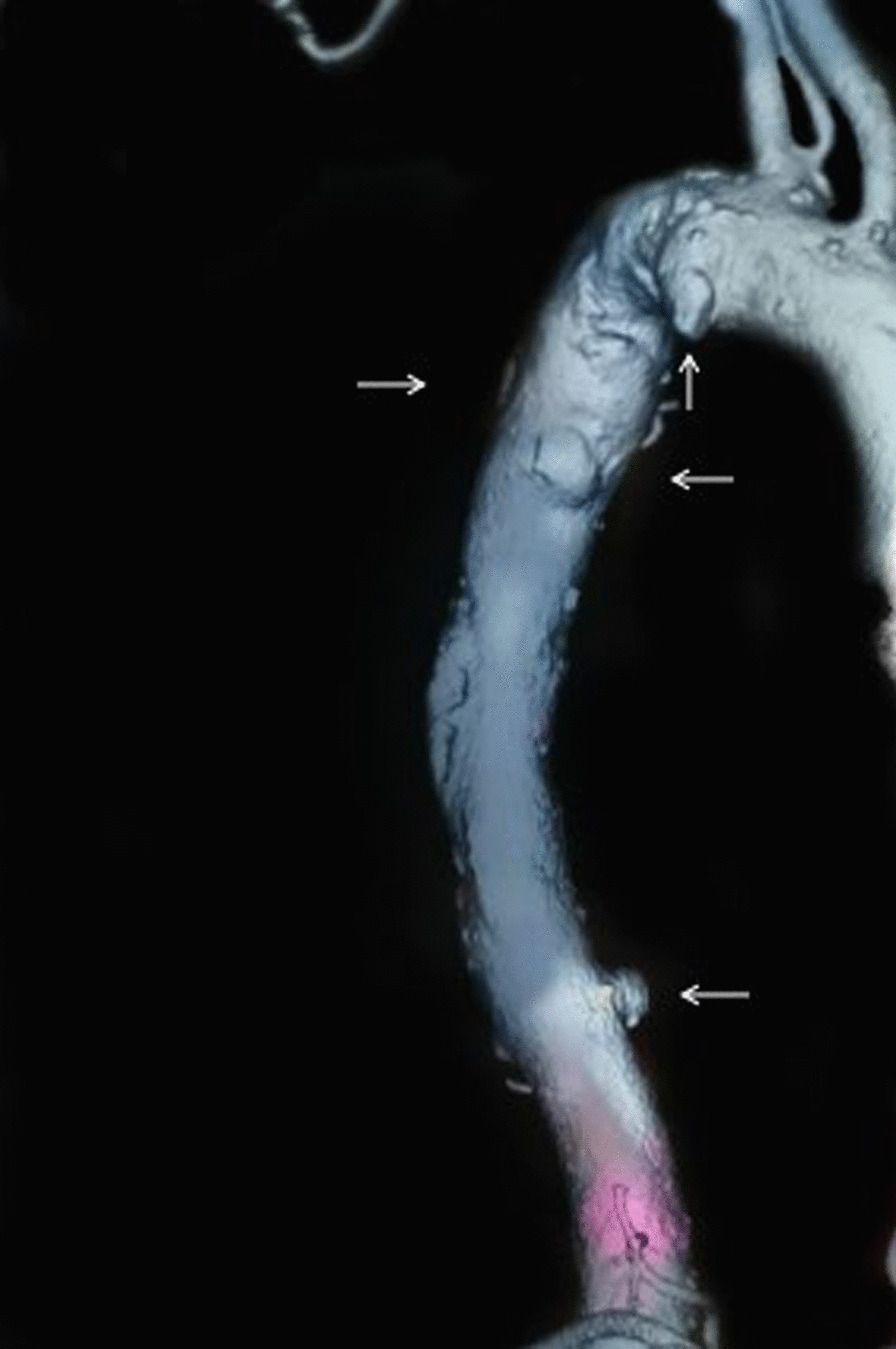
Computed tomography angiography (CTA): Multiple penetrating ulcers in thoracic aorta, partial aneurysm formation
